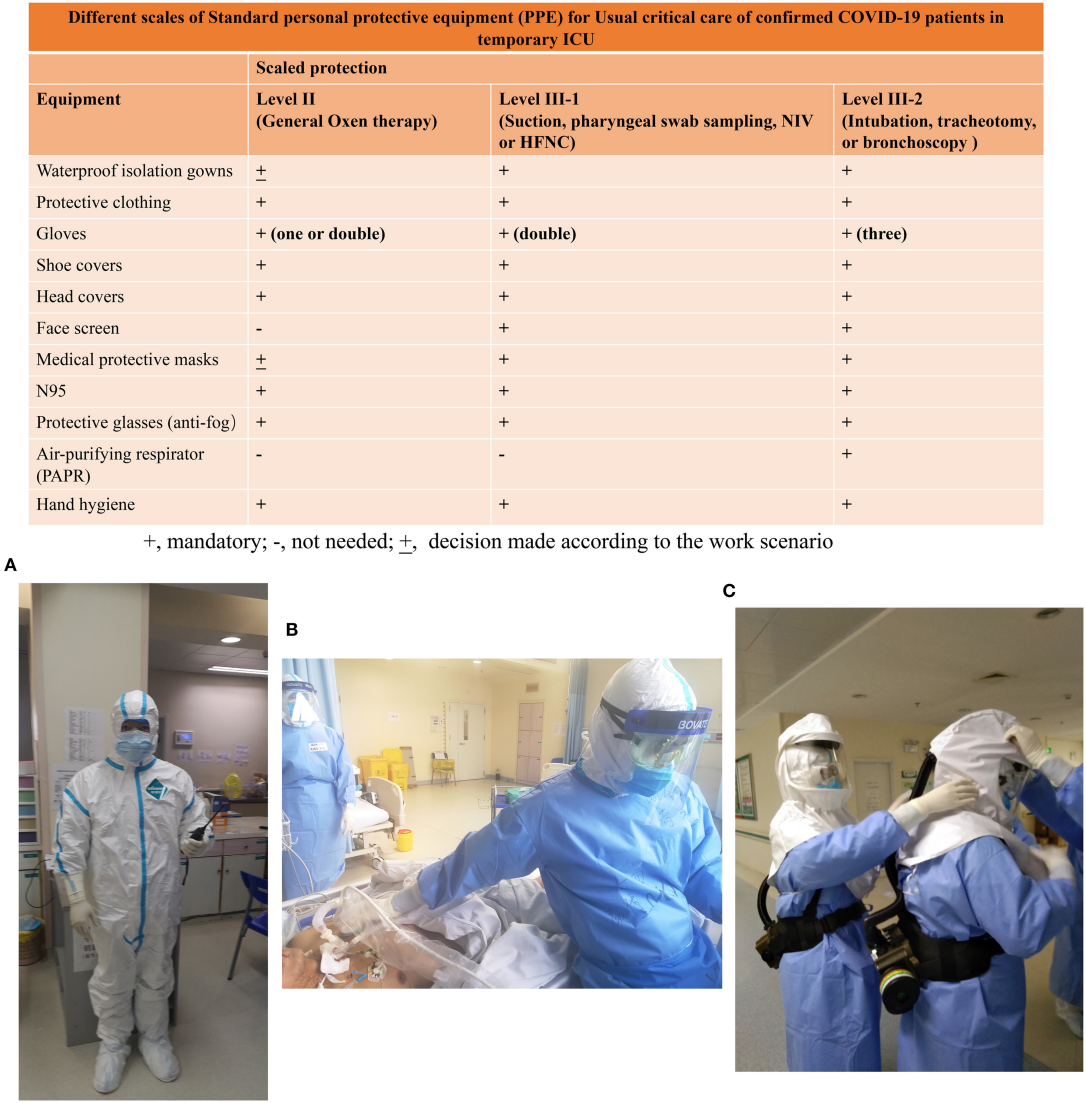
Standard personal protective equipment (PPE) required for normal critical care with COVID-confirmed patients in a temporary ICU. The required standard of PPE is based on the guidelines for the prevention and control of COVID-19 infection in medical institutions laid out by the government in China. Doctors have different scales of PPE to care for severe confirmed patients in different situations. (A) A doctor with level-II PPE at the nurses' station; (B) A doctor with level III-1 PPE for ultrasonography in a temporary ICU; (C) Two doctors with level III-2 PPE preparing for percutaneous tracheostomy in a temporary ICU.
